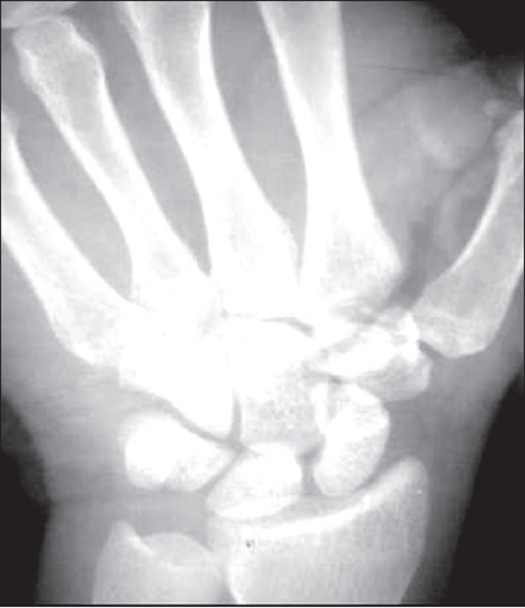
X-rays of wrist (A.P. view) showing fracture of trapezoid
